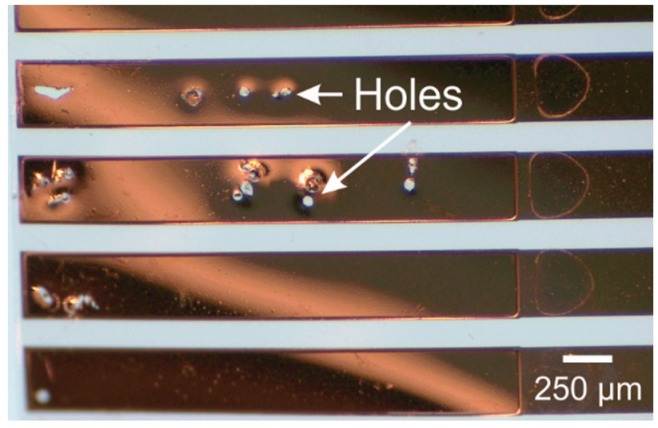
Holes left by gold removal during ultrasonic wire (ball) bonding on a gold thin film on Parylene substrate.
